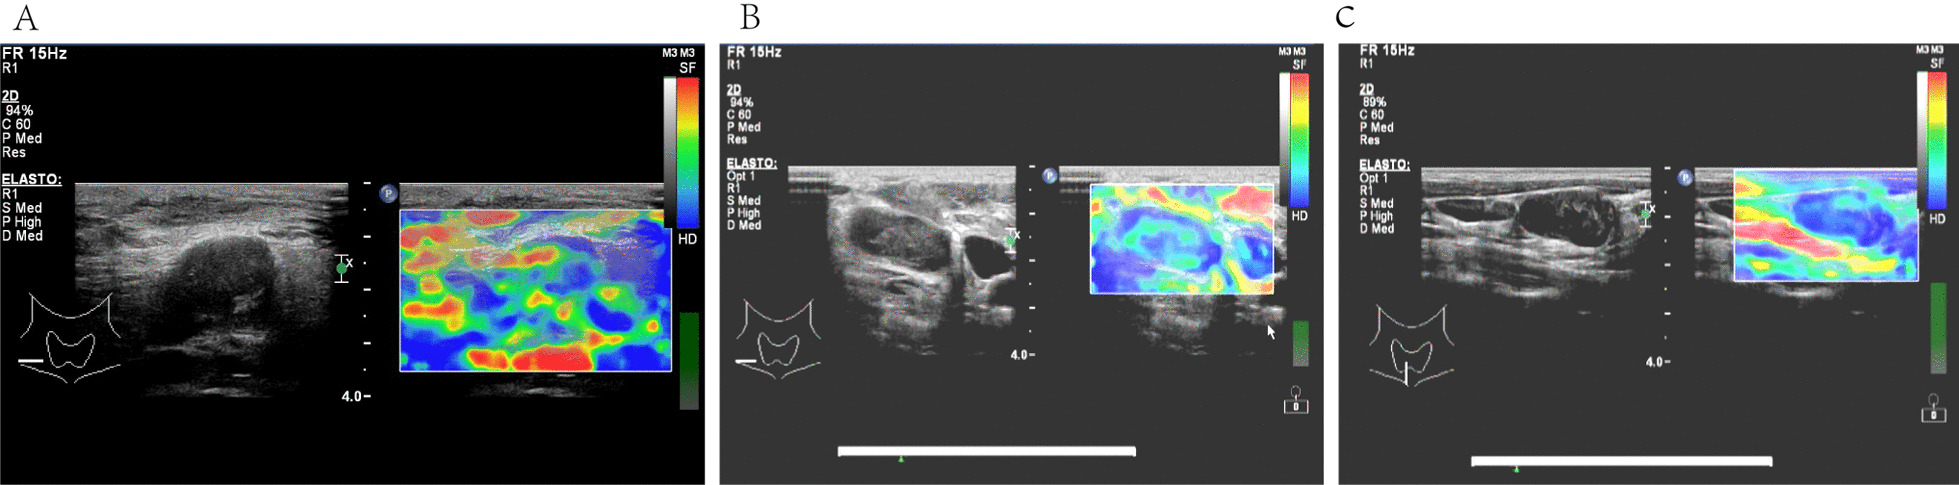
A Ultrasound elastography imaging of tuberculosis in the right cervical lymph nodes, type II. B Ultrasound elastography imaging of tuberculosis in the right cervical lymph nodes, type IV. C Ultrasound elastography imaging of tuberculosis in the right cervical lymph nodes, type V
